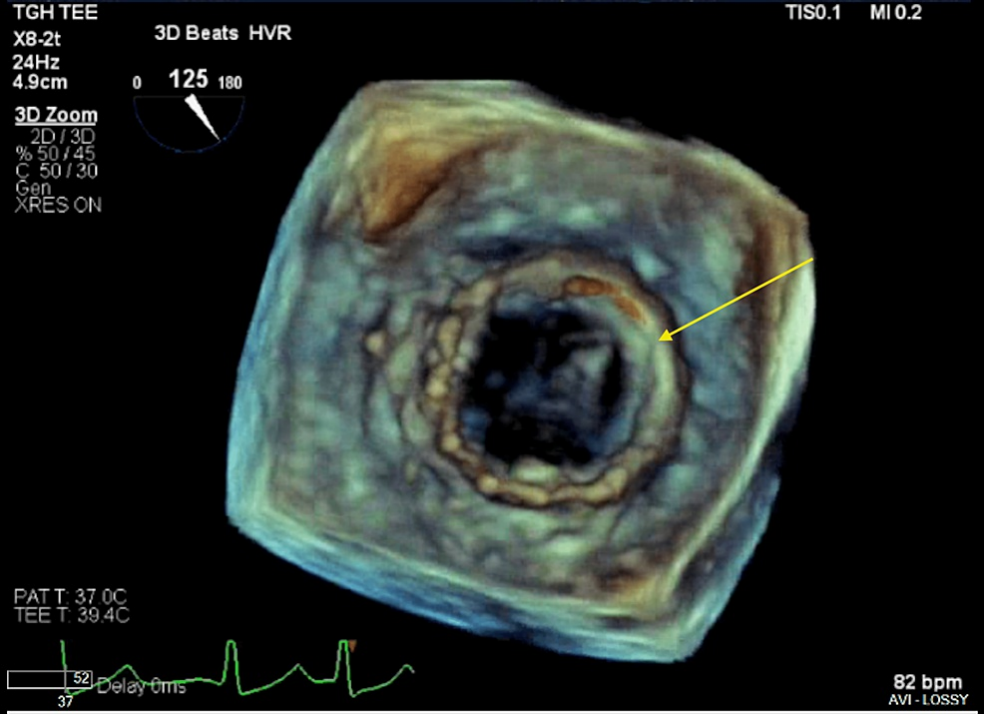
Three-dimensional TEE of the valve-in-valve transcatheter mitral valve replacement (yellow arrow) (surgeon’s view). TEE: Transesophageal Echocardiogram
